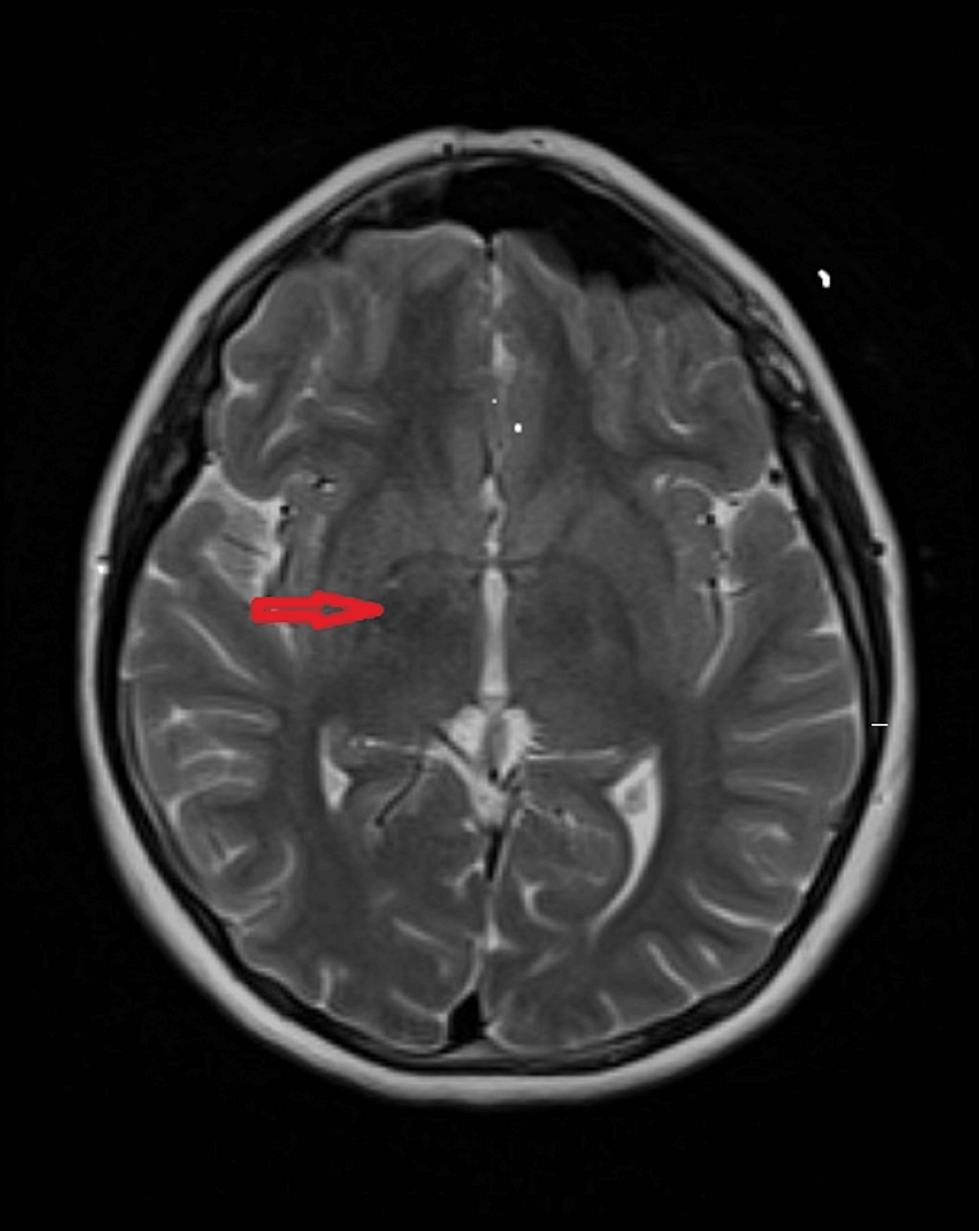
MRI, T2 weighted axial images showing faint collateral vessels (brush sign) in the right basal ganglia (red arrow).
